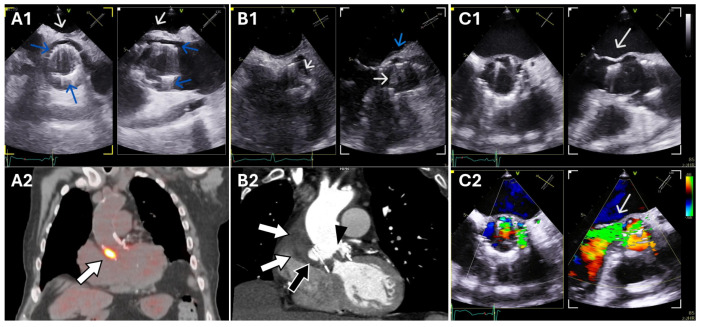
Three cases of PVE in Perceval prosthetic valves. Panel (A1). Pseudo-sinus (blue arrows) and non-specific periprosthetic thickening (white arrow). Panel (A2). [18F]FDG-PET/CT fused image in the same patient showing intense FDG uptake on the prosthetic valve consistent with infection with periprosthetic involvement. Panel (B1). Leaflet thickening suggestive of vegetations (white arrows) and posterior periannular thickening (blue arrow); panel (B2). Corresponding cardiac CT confirmed vegetations (arrowhead) associated with periprosthetic and periaortic abscess (white arrows and black arrow). Panels (C1,C2). Perceval prosthesis dehiscence and severe paravalvular regurgitation (arrow). PVE, prosthetic valve endocarditis; [18F]FDG-PET/CT, 18F-Fluorodeoxyglucose Positron Emission Tomography/Computed Tomography; CT, Computed Tomography.
